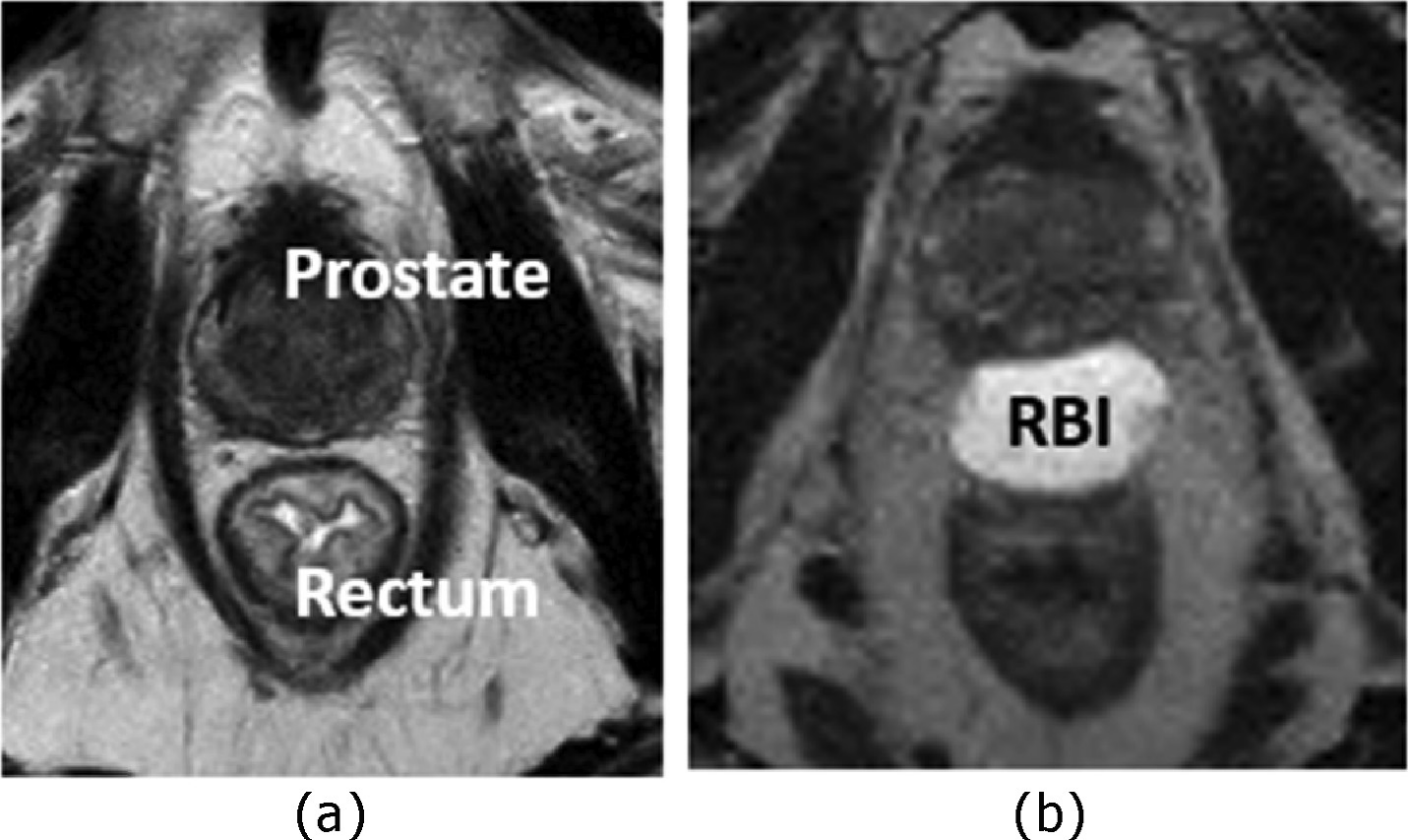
Axial T2-weighted MRI of a patient with a RBI before (a) and after implantation (b). *Abbreviation*: MRI = Magnetic Resonance Image; RBI = Rectal Balloon Implant.
